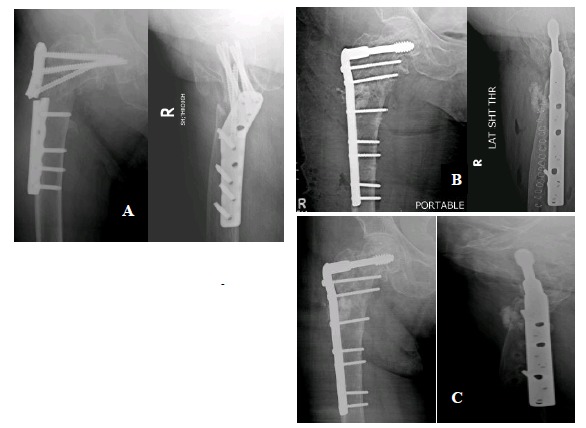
A) Varus collapse with PF-plate 18 months post-op, B) Immediate post-op radiographs after revision with DCS, C) Radiographs taken 6 months post revision showing good healing.
